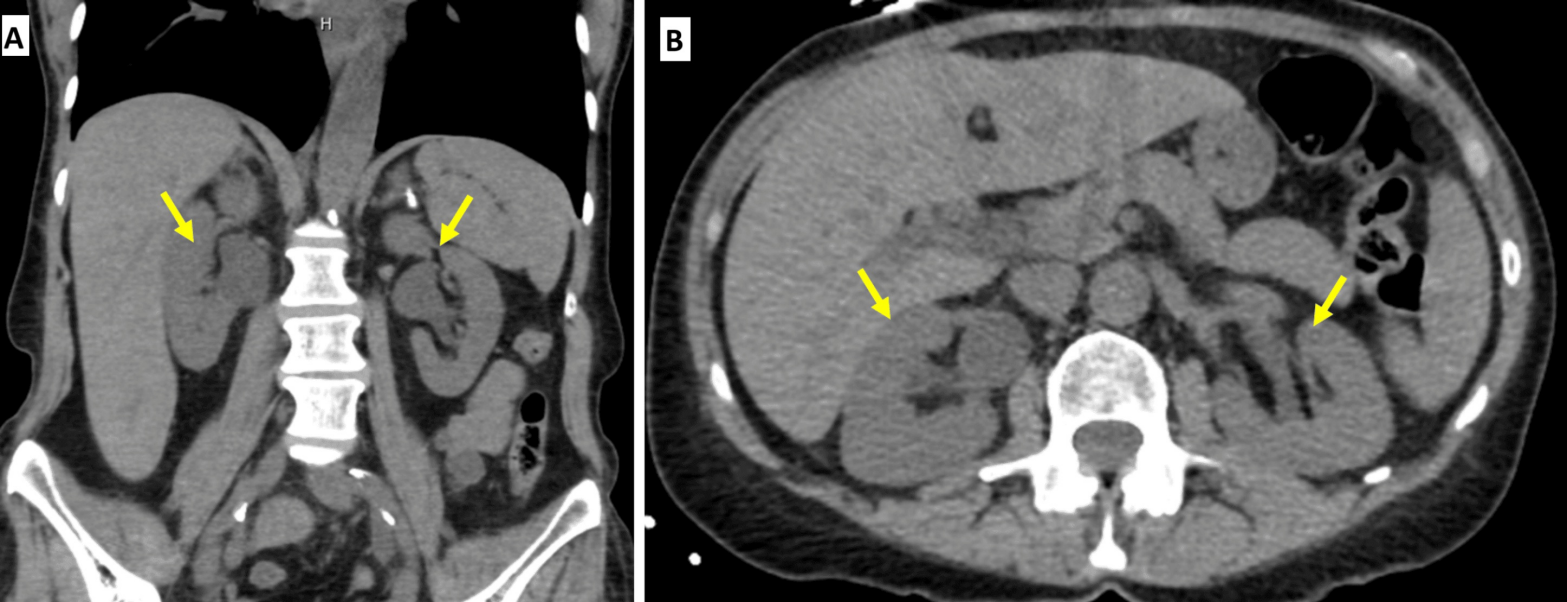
CT abdomen and pelvis show bilateral hydroureteronephrosis (yellow arrows).
